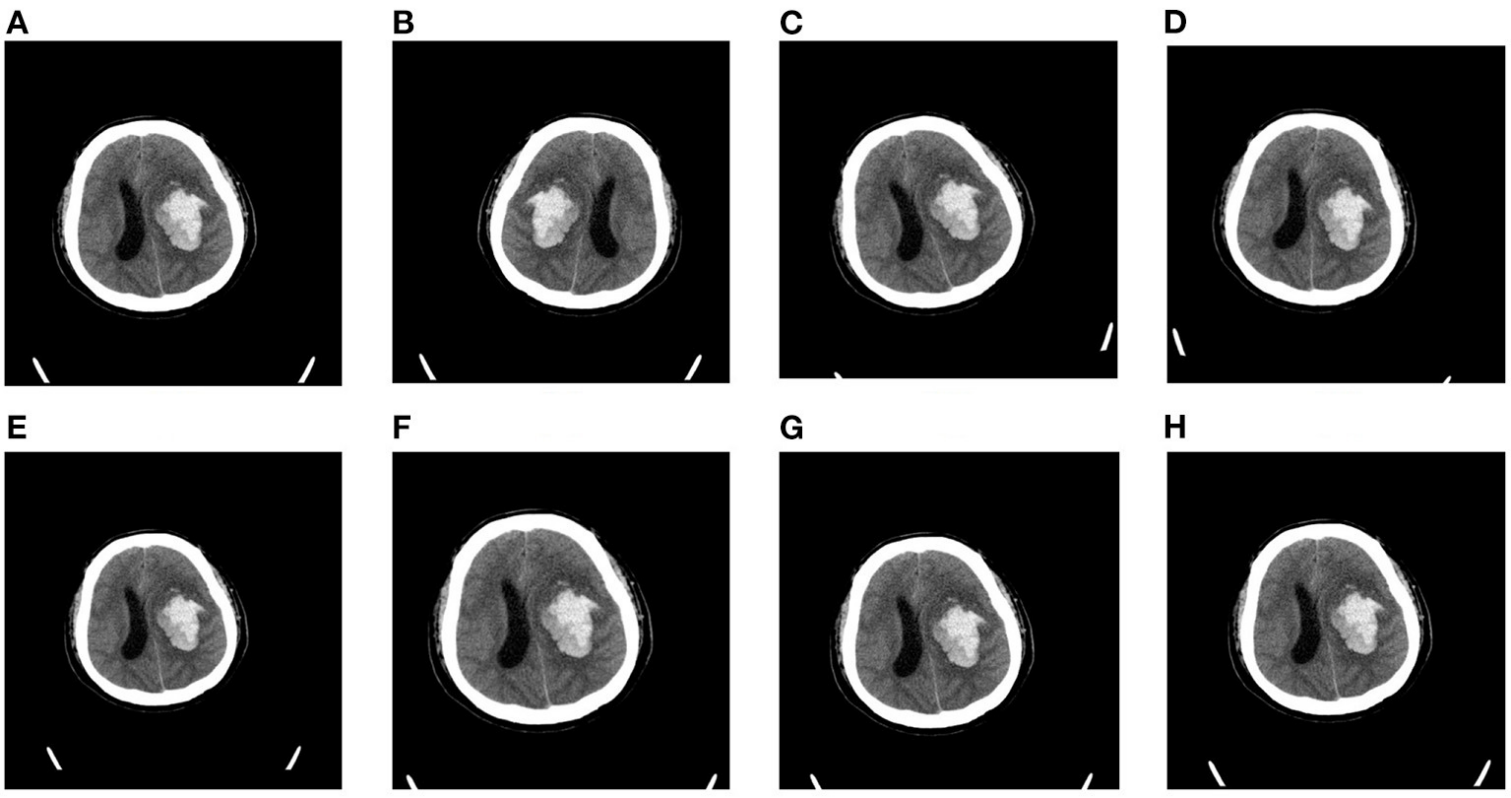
Random augmentations for CT slices: (A) original slice, (B) left-right flipping, (C) rotation (10°, (D) rotation (−10°, (E) scaling (0.9), (F) scaling (1.1), (G) shifting (right), and (H) shifting (down).
